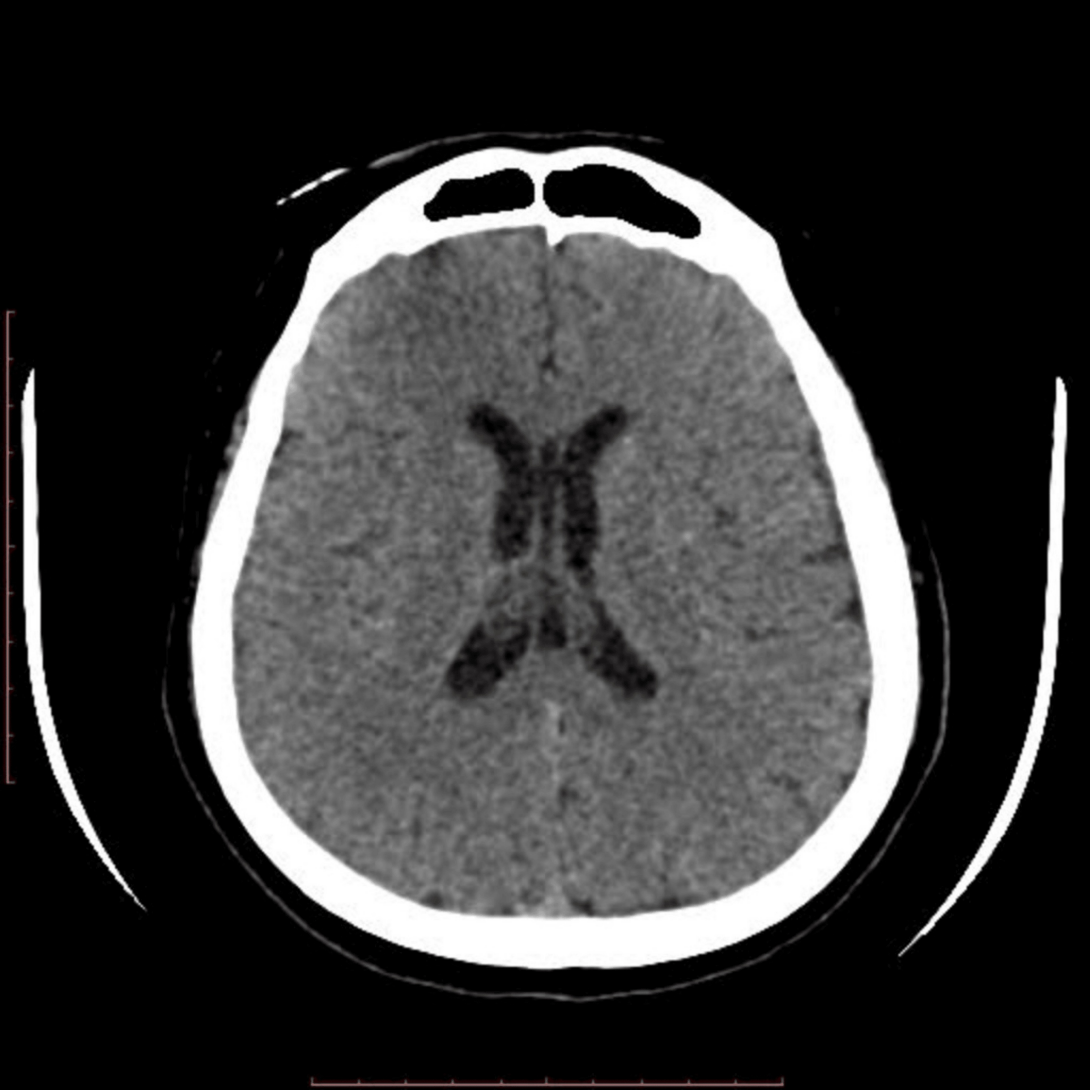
CT brain shows poor gray-white matter differentiation with sulcal effacement and mildly dilated ventricles, suggestive of cerebral edema and communicating hydrocephalus.
